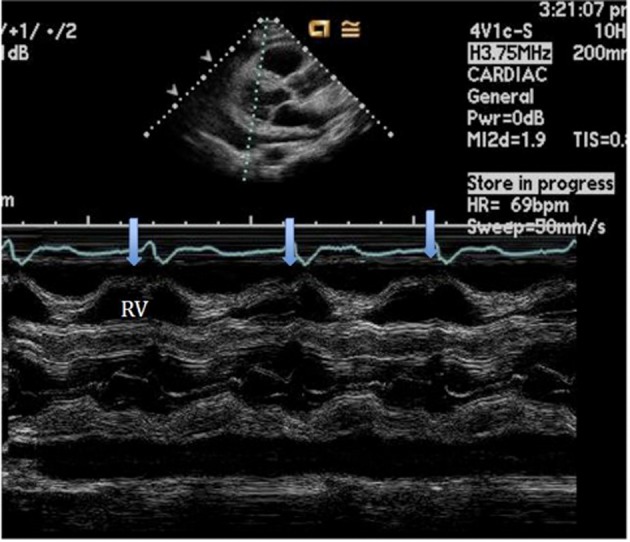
M-mode of the parasternal long axis view establishing the diagnosis of cardiac tamponade showing the collapse of the RV during diastole.
